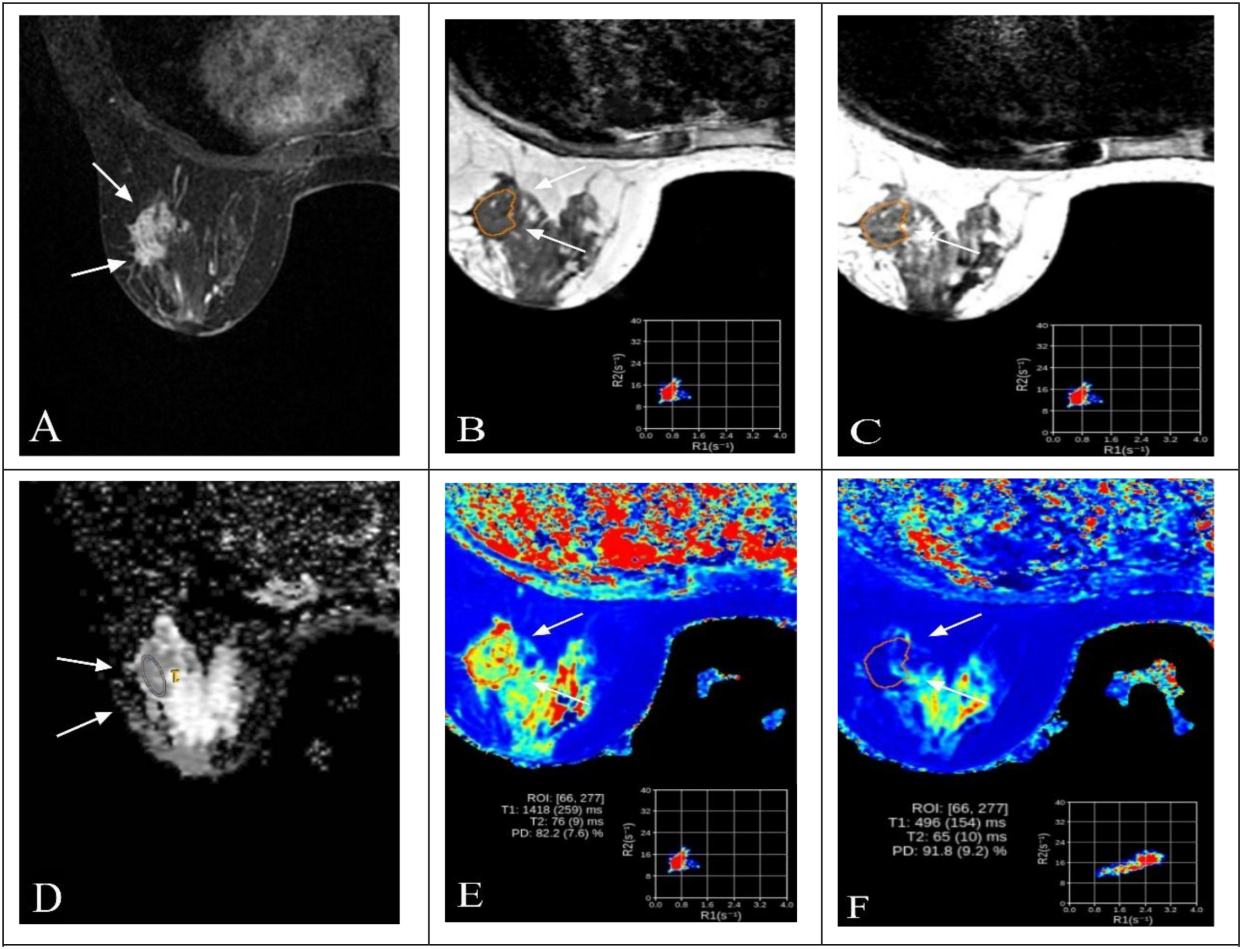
Representative multiparametric MRI findings in a 63-year-old woman with invasive breast cancer and HER2 overexpression (IHC 3+). (A) DCE-MRI showing a heterogeneously enhancing lesion in the right breast (arrows). (B, C) SyMRI parametric maps (T1-pre and T2-pre) with ROI placement. (D) ADC map from MUSE-DWI demonstrating restricted diffusion (arrows). (E) Pre-contrast SyMRI T1 mapping (T1-pre) with quantitative measurement. (F) Post-contrast SyMRI T1 mapping (T1-Gd) showing shortened relaxation time within the lesion.
